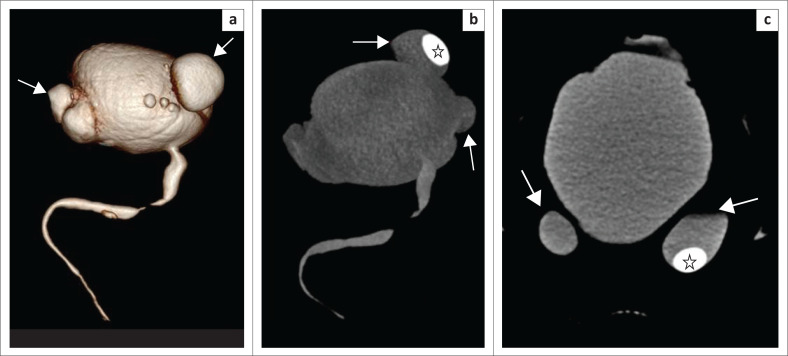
(a) Volume rendered technique, (b) maximum intensity projection and (c) axial multiplanar reformatted (MPR) images of multidetector computed tomographic urethrography. All the images are of the same patient, showing multiple bladder diverticula (arrows). A calculus (asterisks) is noted within the diverticulum on coronal and axial MPR images.
